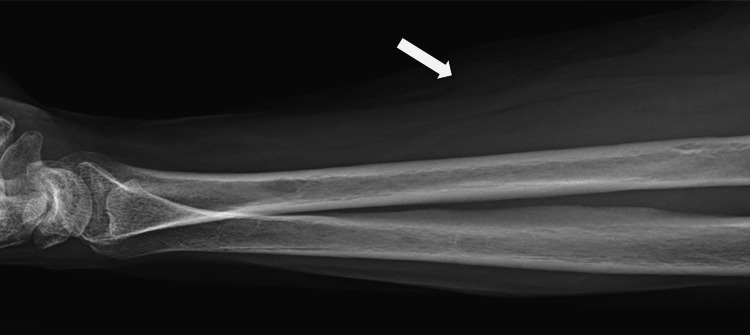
X-ray of the right forearm Soft tissue density along the mid-volar forearm (white arrow).
